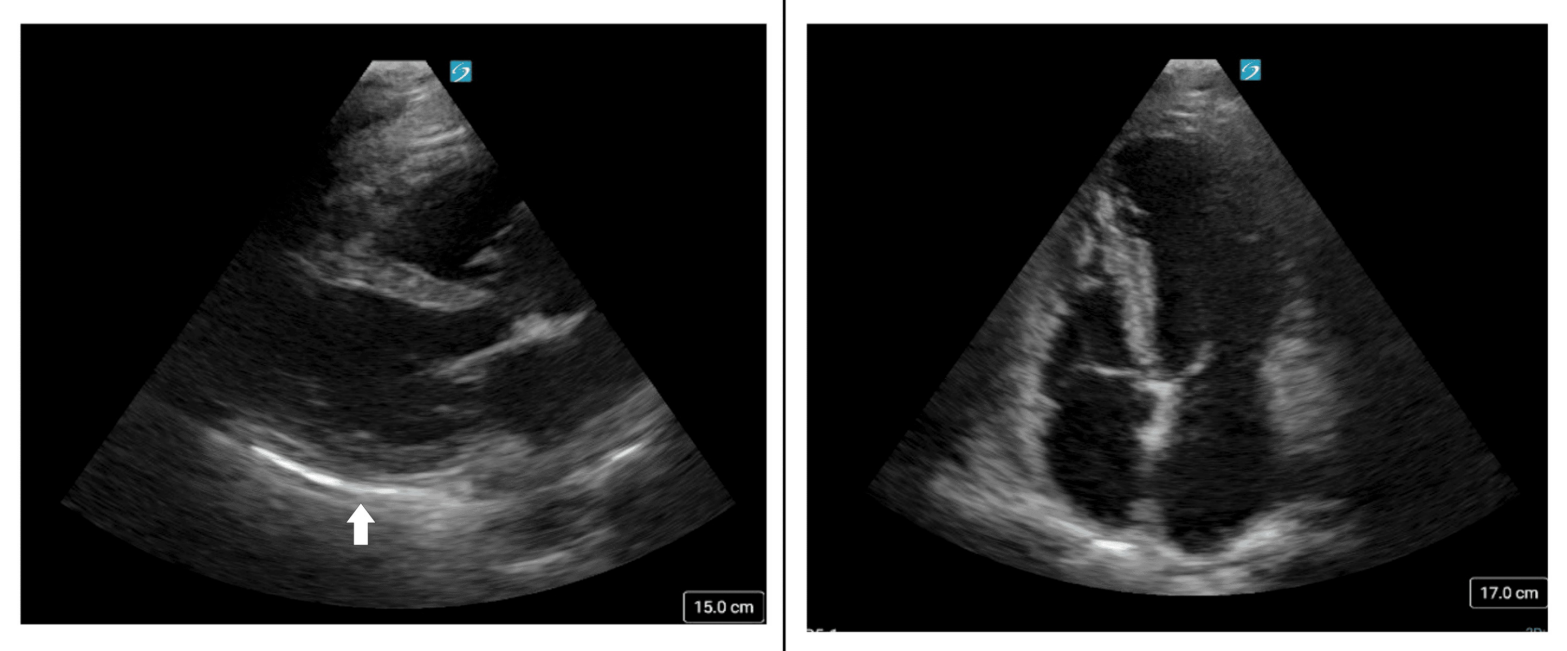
Cardiac point-of-care ultrasound showing the parasternal long-axis (left) and apical four-chamber (right) views. A trace pericardial effusion is seen (left, arrow). No wall-motion abnormality, valvular pathology, or reduction in ejection fraction was observed.
